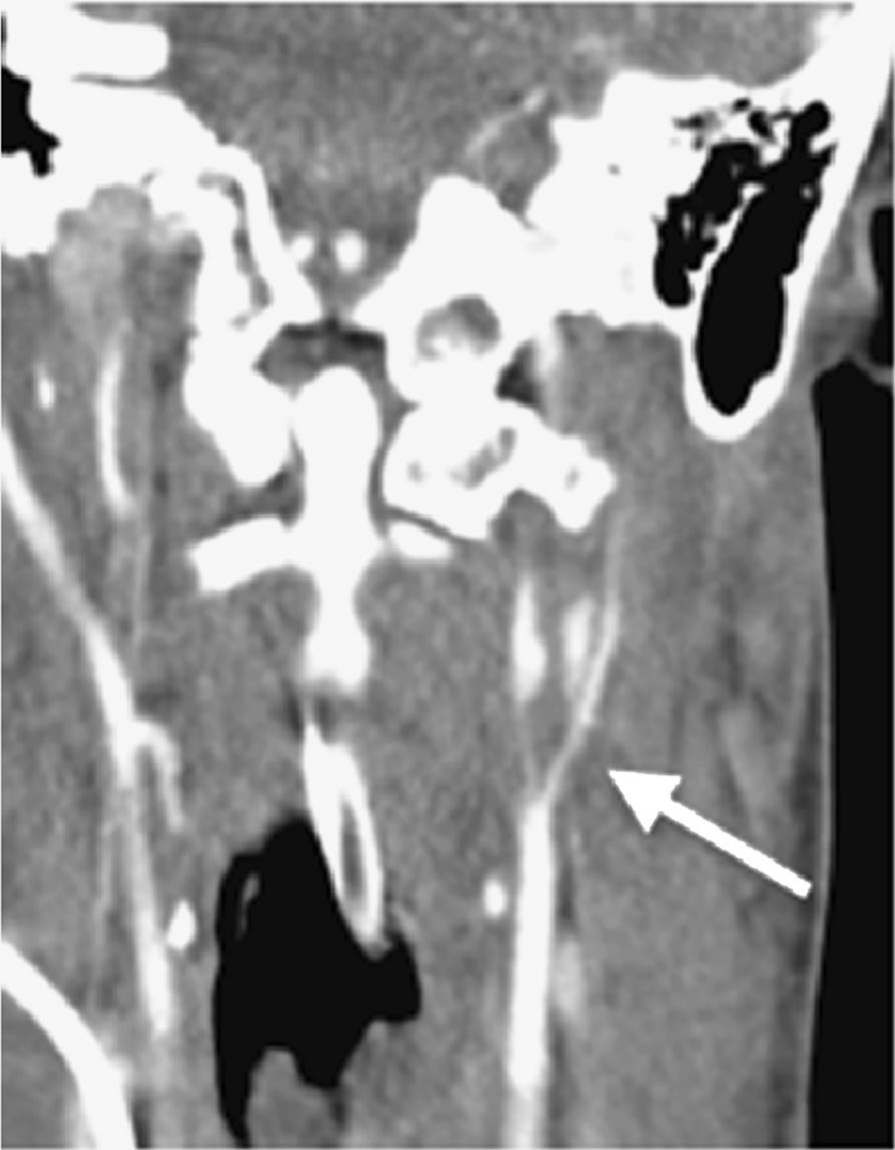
Second CT scan after ICU admission with coronal reconstructions of the bilateral ICA dissection. “String sign” indicated with *an arrow*.
